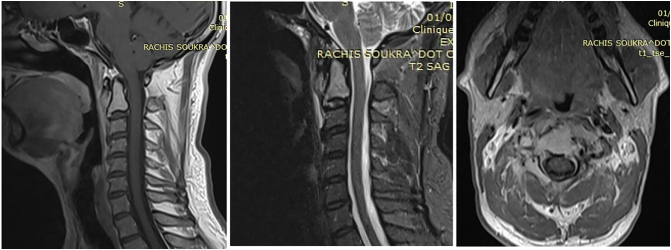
MRI images showing an infiltrative process involving the axis vertebral body, as well as the odontoid process, with invasion of the right side of the posterior arch and extension into the soft tissues.
